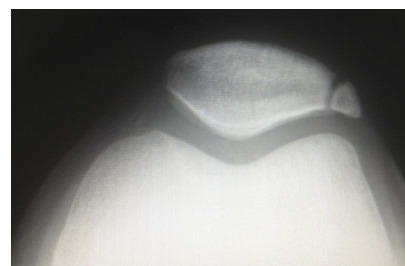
Initial radiograph in a patient with anterior knee pain following fall onto left knee, showing bipartite patella with no bony abnormalities.
